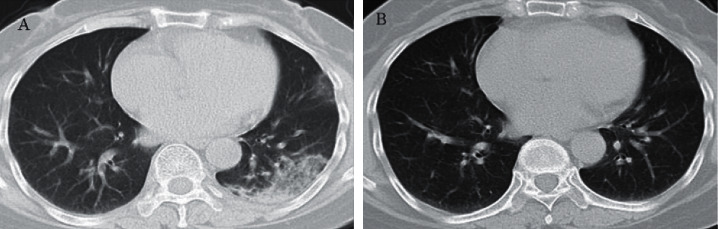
(a) At discharge, lung CT showed high-density and patchy shadows in the left lung with blurred edges. (b) The lung lesions were absorbed by reexamination three months after discharge.
